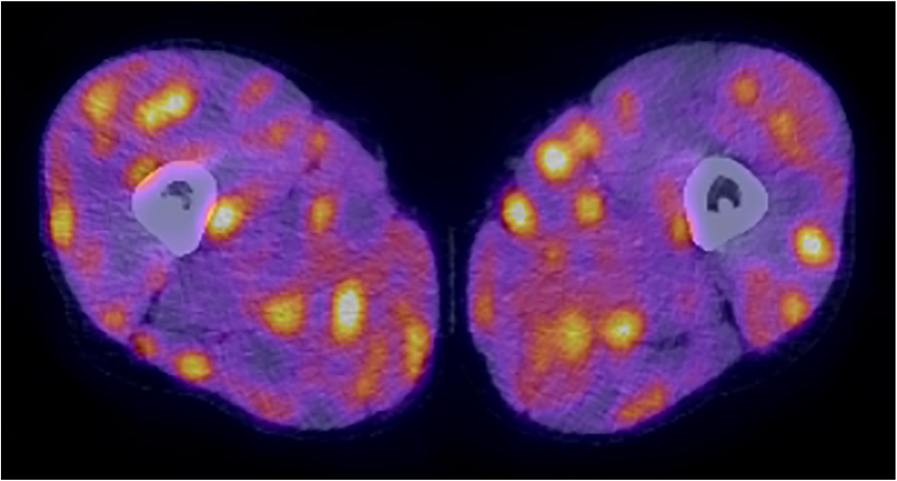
A positron emission tomography/computed tomography examination of the upper thighs 1 year after initial presentation. An axial positron emission tomography/computed tomography image demarks diffuse bilateral distribution of spots with increased fludeoxyglucose uptake of all muscles of the upper thighs
